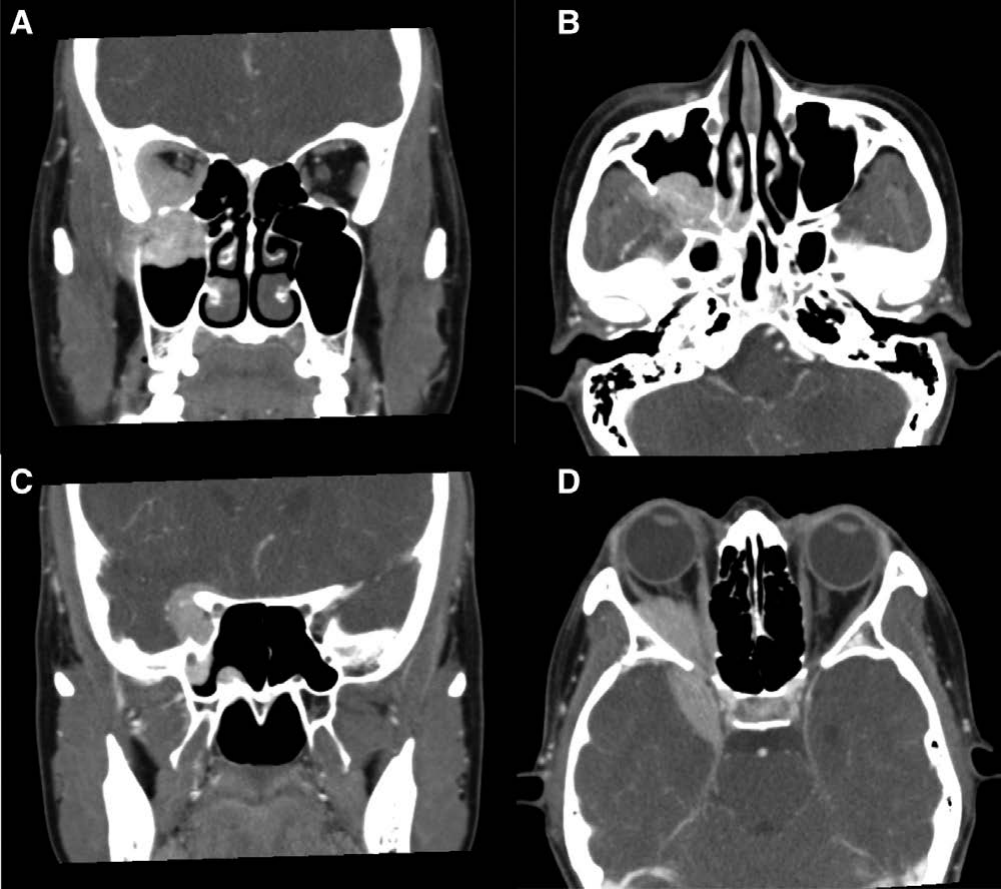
Orbital computed tomography scans show a well-enhancing tumor. (A) Axial and (B) coronal views of the orbital mass in the retrobulbar area extending into the right maxillary sinus. (C) Axial and (D) coronal views of the same mass extending into the right middle cranial fossa through the superior orbital fissure and invading the adjacent brain tissue.
